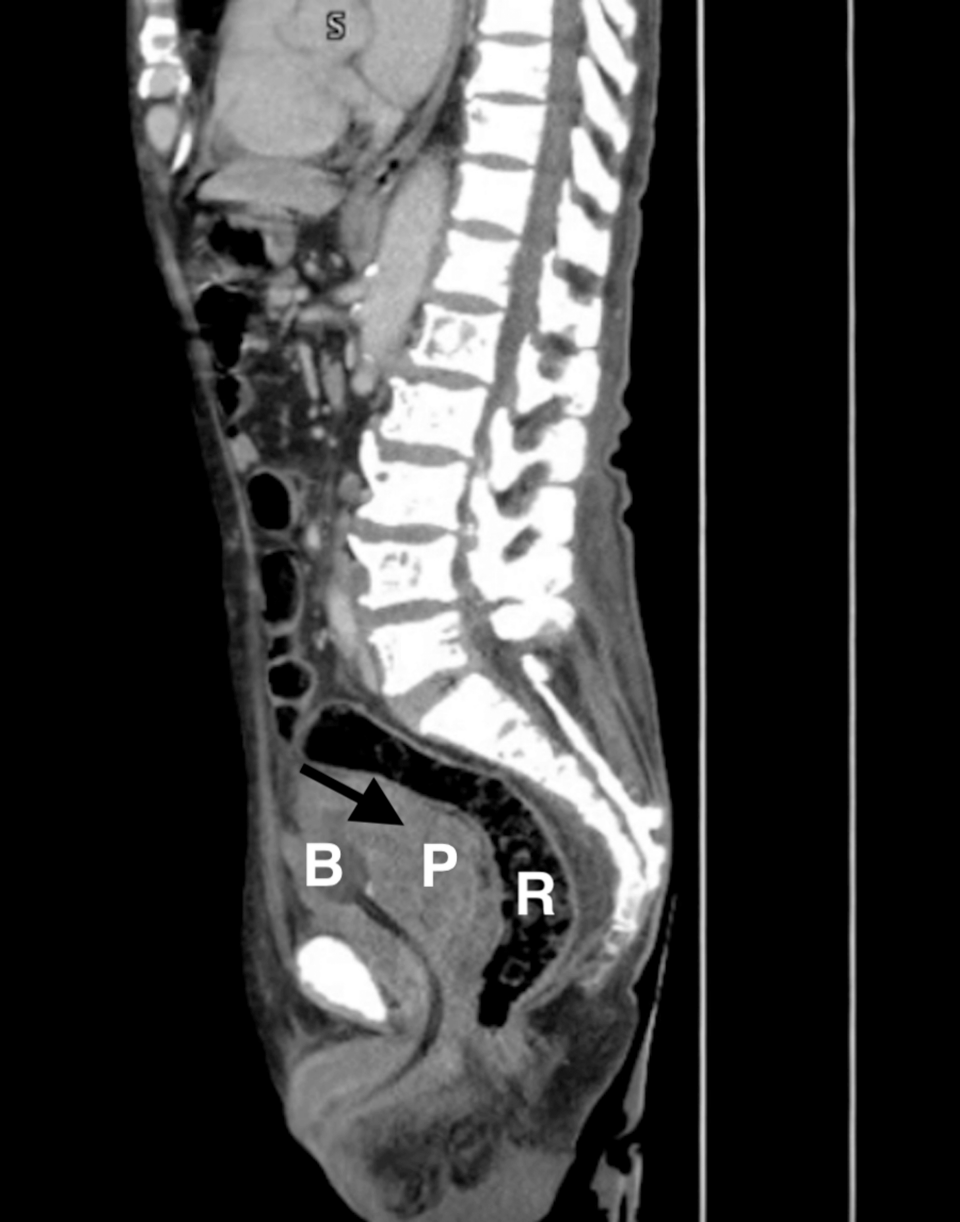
Contrast-enhanced abdominopelvic computed tomography (CT) in the sagittal plane demonstrates a markedly enlarged and heterogeneous prostate gland (arrow). There is loss of the normal fat planes between the prostate (P), bladder (B), and rectum (R), indicating direct tumor invasion into adjacent structures.
